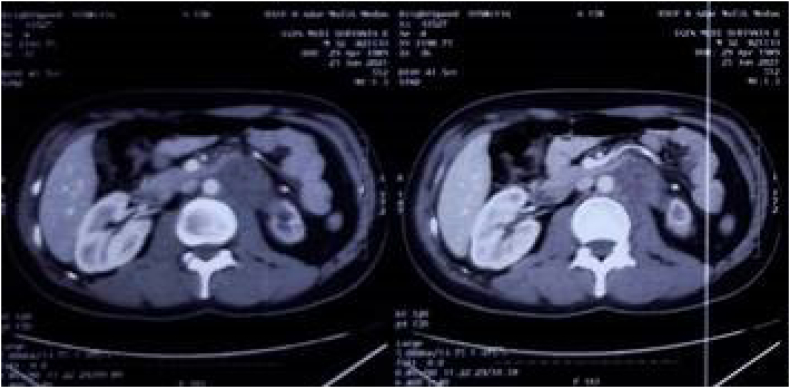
CT scan with contrast 6 months after BEP administration.
